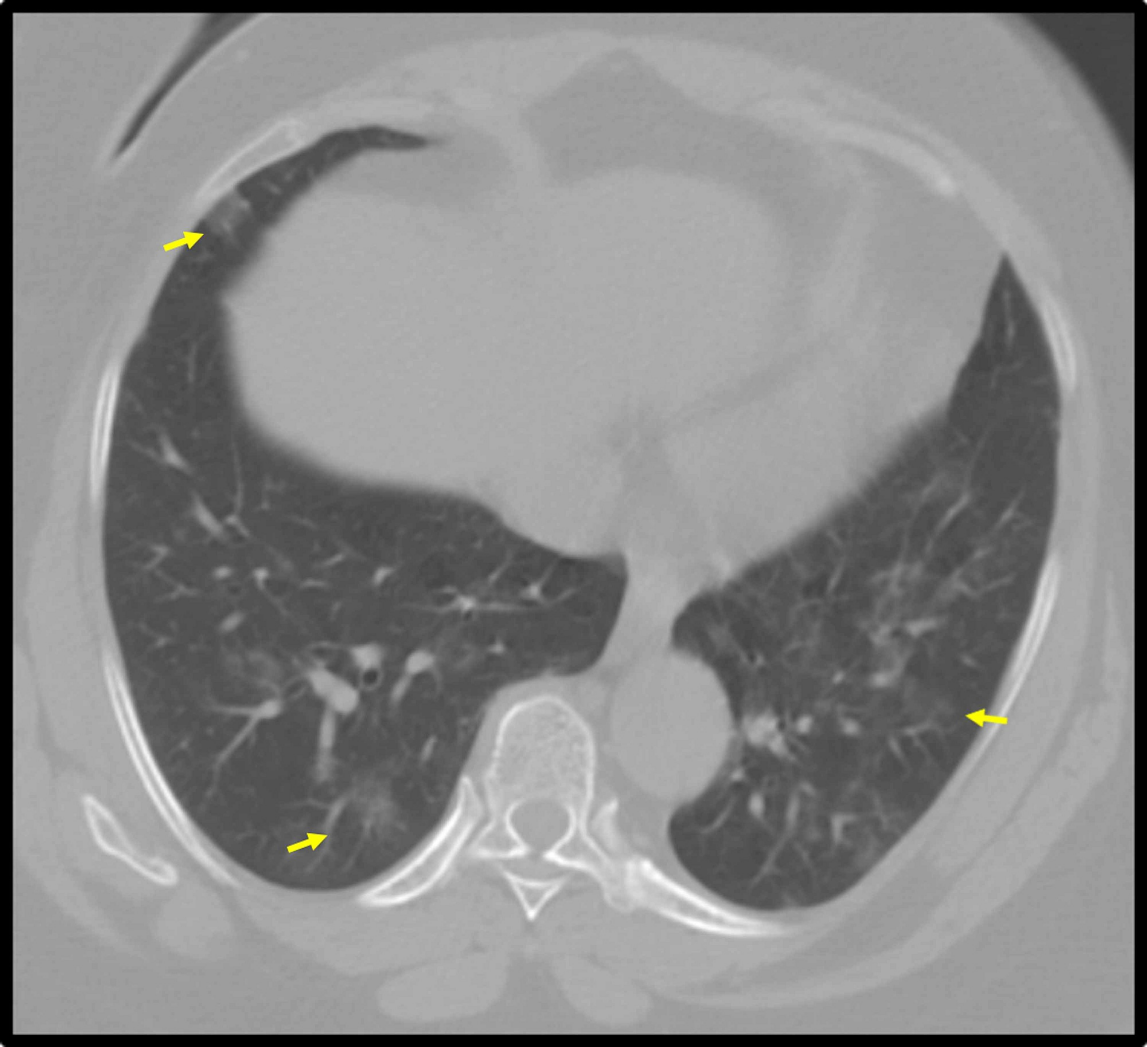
Axial image from a CT of the abdomen and pelvis without contrast through the lung bases The image shows peripherally predominant ground-glass opacities bilaterally (yellow arrows) CT: computed tomography
